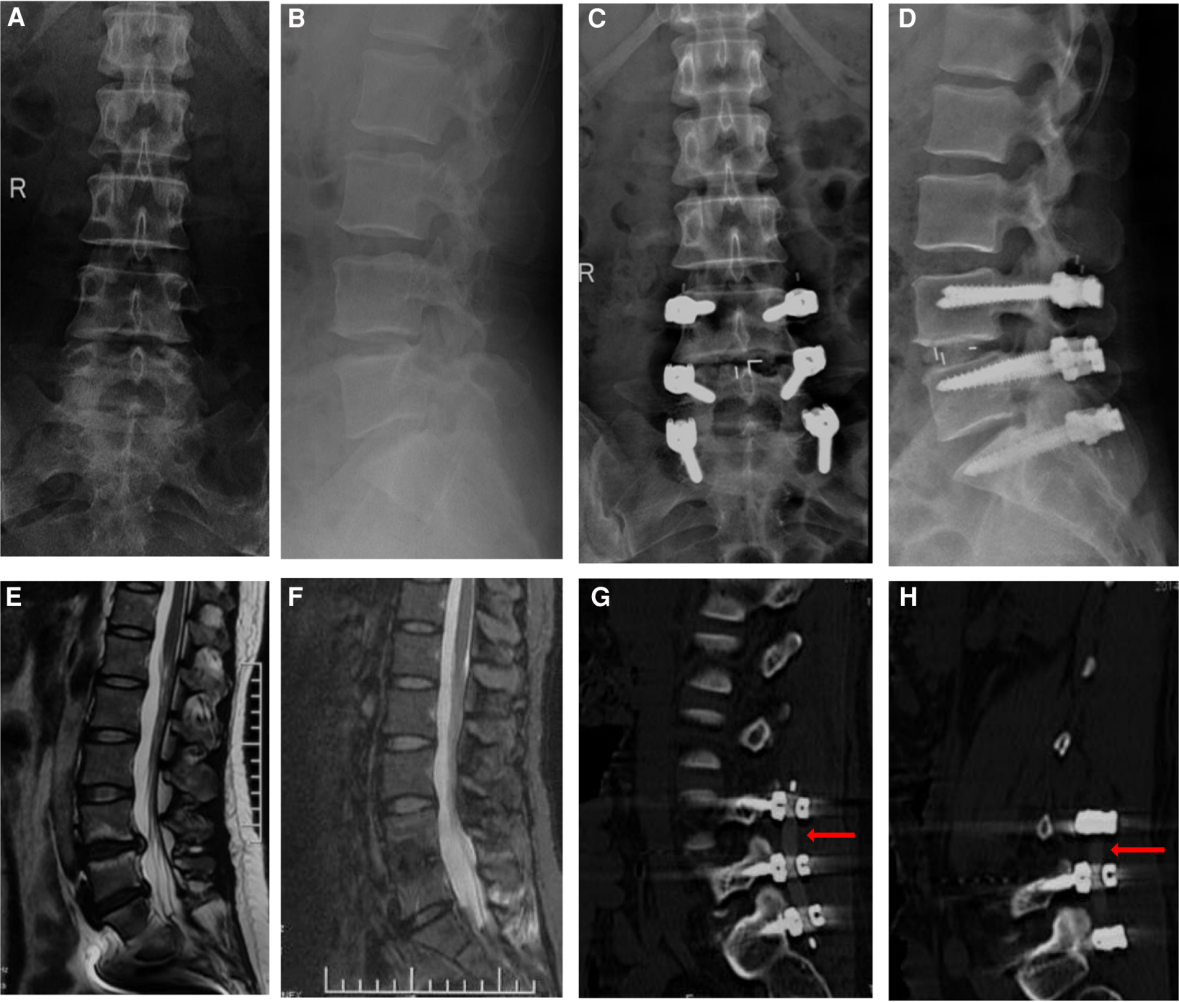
A 45 years female patient diagnosed with lumbar spinal stenosis underwent posterior PEEK rods hybrid surgery with fusion procedure at the L4/5 level and non-fusion procedure at the L5/S1 level. Preoperative anterior-posterior and lateral fluoroscopy was showed in (A,B), while postoperative anterior-posterior and lateral fluoroscopy at the final follow-up was showed in (C,D). Compared preoperative sagittal MRI scans (E) with sagittal MRI scans at the final follow-up (F), it was found that intervertebral disc degeneration improved from Grade 4 to Grade 3 according to Pfirrmann Classification. The CT reconstruction confirmed that the bilateral PEEK rods were intact at the final follow-up (G,H).
